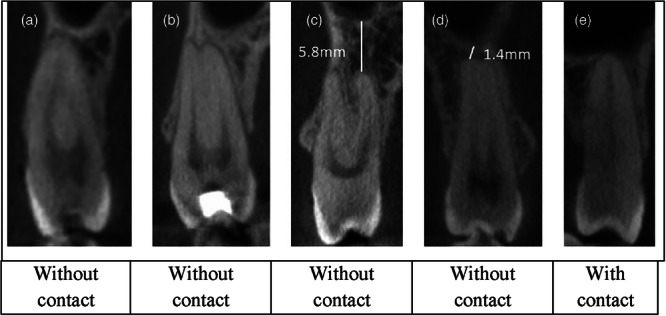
An example of cone‐beam computed tomography images for evaluating the contact between teeth and the sinus (a–d) without contact and (e) with contact.
